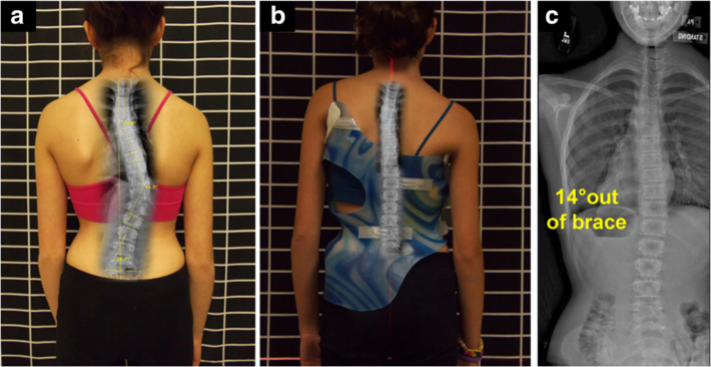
WCR out-of-brace correction from 41 degrees to 14 degrees Cobb angle. a: The clinical presentation of the patient with a right thoracic curve of 41 degrees Cobb angle. b: In-brace X-ray of patient with Cheneau type brace 18 hours after initial fitting. The right thoracic curve reduced in-brace from 41 degrees to 5 degrees Cobb angle and the upper thoracic curve reduced in-brace from approximately 25 degrees Cobb angle to 10 degrees Cobb angle. c: Out-of-brace X-ray showed a reduction in the major curve from 41 degrees prebrace to 14 degrees out-of-brace.
